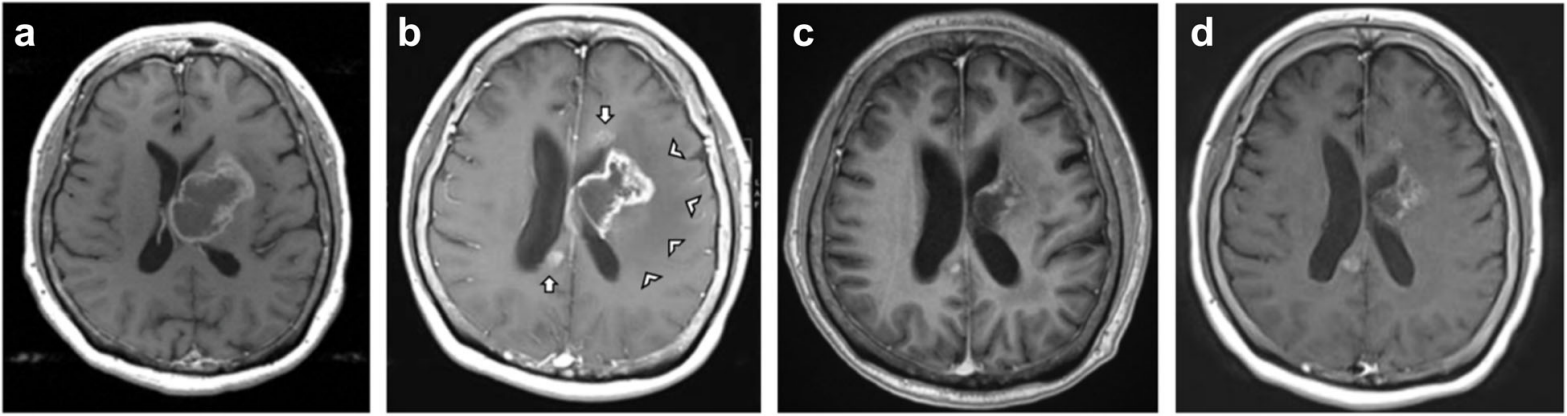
T1 axial postcontrast MRI images a Initial MRI showing left basal ganglia tumor with swollen left thalamus, insula cortex, and hippocampus suggesting multifocal GBM. b Follow up MRI 4 months after primary diagnosis showed an irregular 4.5 cm × 4.0 cm × 5.7 cm (AP x TR x CC) residual tumor. Extensive vasogenic oedema of left frontal lobe was seen extending to left insula, left hippocampal gyrus and body of corpus callosum (arrowhead). Additionally, a smaller ~ 1 cm enhancing lesions are seen, suggestive of local tumor extensions (arrowed). c MRI evaluation at fifth cycle of bevacizumab, carboplatin, and low-dose olaparib revealed shrinkage of left basal ganglia tumor compared to (b). d (2nd craniotomy pre-op) MRI for tumor stereotaxy and surgical planning showed an irregular 1.5 cm × 3 cm tumor mass with predominant peripheral enhancements and central necrosis at left basal ganglia, compressing on the body of the left lateral ventricle
